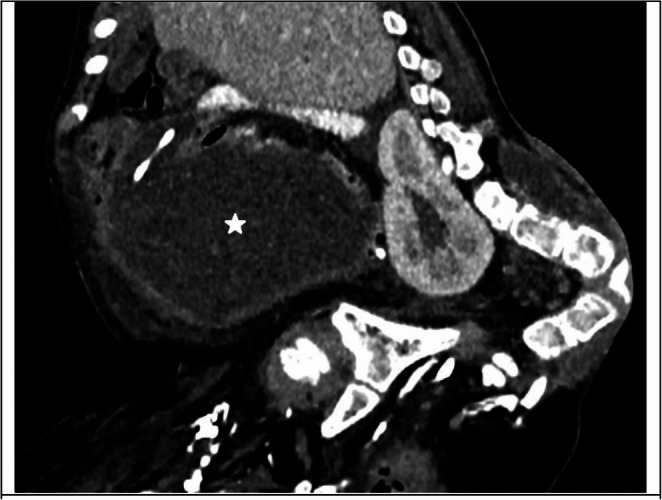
An abdominal CT scan of a 9-year-old male patient (patient no. 3) demonstrates an abdominal pseudocyst (asterisk) containing the distal catheter. The patient has sacral agenesis and severe lumbar kyphosis, with a history of both prior abdominal surgery and multiple revision surgeries. In this case, the peritoneal cavity could not be salvaged, and the patient ultimately underwent conversion to a VA shunt
